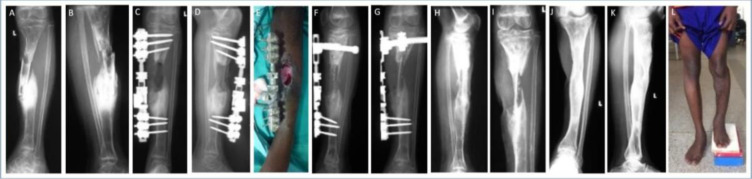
A 12-year-old boy presented with complicated tibial osteomyelitis with an exposed sequestrum, pathological fracture as well as bone and soft tissue loss. Radiographs showed sequestration of the central shaft of the tibia (a, b). Sequestrectomy was performed, and the tibia was stabilized with an external fixator. A proximally based hemisoleus flap was transferred 2 weeks after the debridement (c, d, e). Three months later, a tibial cortex strut was inserted (f, g). The fixator was removed 6 weeks later and replaced with a long-leg cast. Radiographs are shown at 4 months after grafting (h, i). Seven years later, they showed full graft integration, no infection recurrence and moderate varus of the ankle. (j, k, l) The patient reported moderate pain in the knee and ankle joints after long walks. The LLD was 7 cm, but the patient refused any shoe raise. Proposal for further surgery for lengthening was put on hold by the family.
